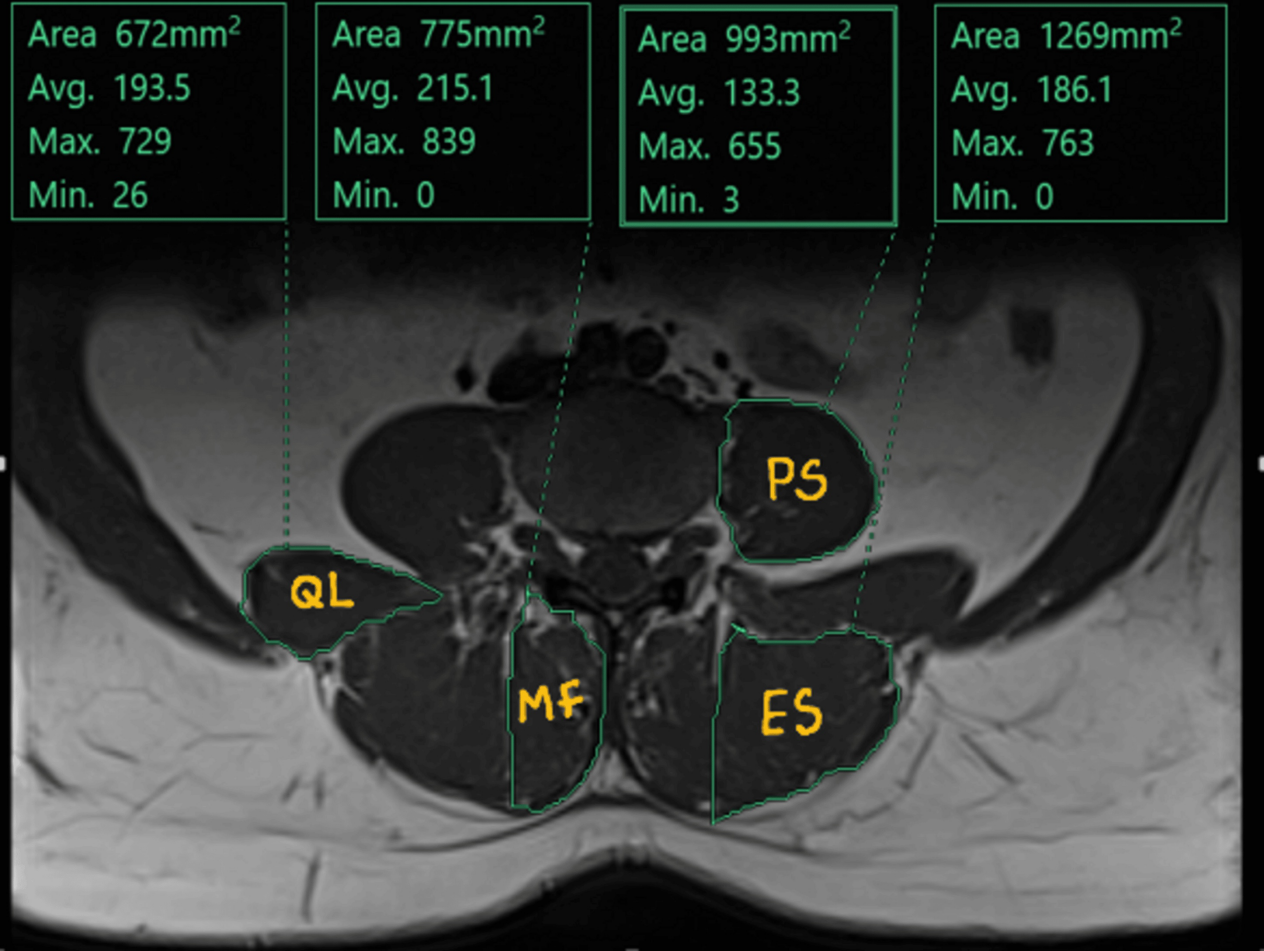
MRI axial T2-weighted image demonstrating the cross-sectional area (cm²) of paraspinal muscles—erector spinae, multifidus, psoas major, and quadratus lumborum—at the level of the L4 superior endplate. MRI: Magnetic Resonance Imaging; L4: Fourth Lumbar Vertebra; ES: Erector Spinae; MF: Multifidus; PS: Psoas Major; QL: Quadratus Lumborum
